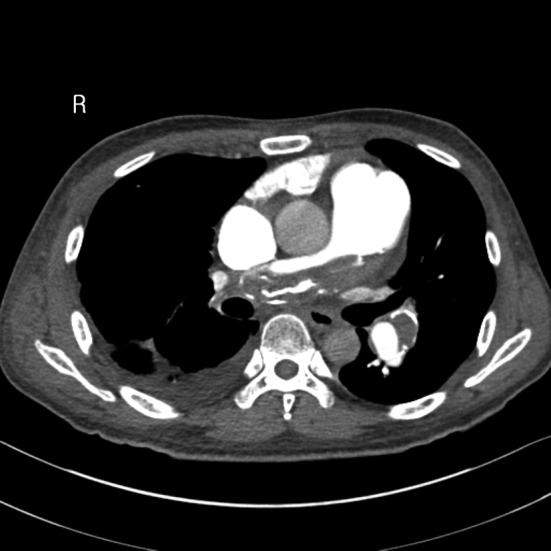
Chest computed tomography showed diffused thrombus in the central and both main pulmonary arteries.
